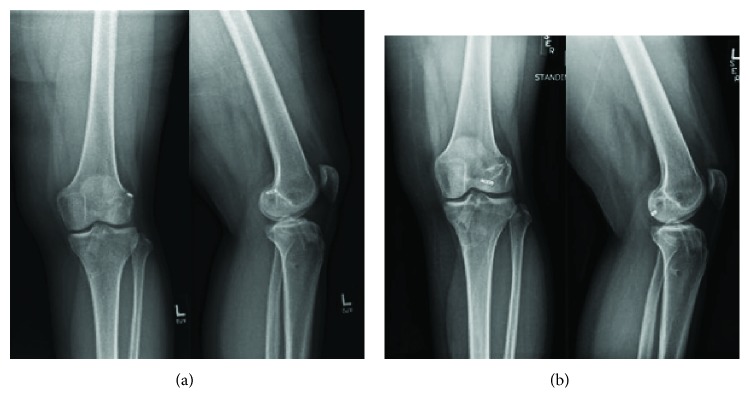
(a) Eighteen-month postoperative radiographs of the left knee performed as a screening examination due to pain following minor knee trauma. AP: lateral and notch views demonstrate expected postoperative changes from ACL reconstruction with well-positioned femoral tightrope button. (b) Two-year postoperative radiographs of the left knee performed due to new onset of symptoms in the posterior knee. There is evidence of interval migration of the TightRope button into the posterolateral joint space.
